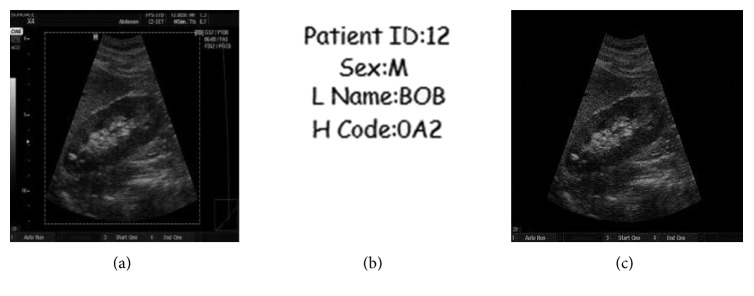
(a) Original ultrasound image. (b) Watermark logo. (c) Watermarked image. Hash of the image: a16c4d371f512de40f836428ea2541fe.
